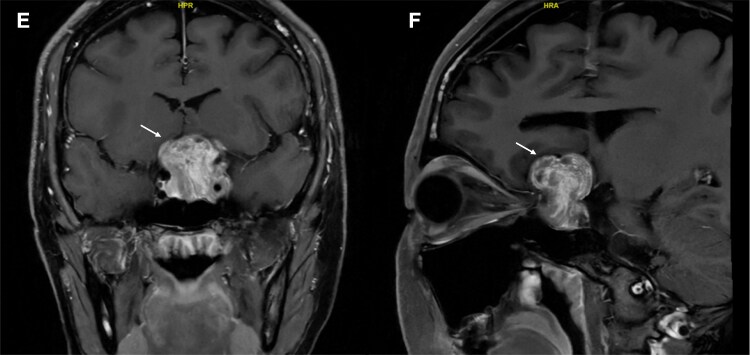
(E) Coronal section of T1-weighted postcontrast MRI images of the brain preoperative. (F) Sagittal section of T1-weighted postcontrast MRI images of the brain preoperative. Abbreviation: MRI, magnetic resonance imaging.
